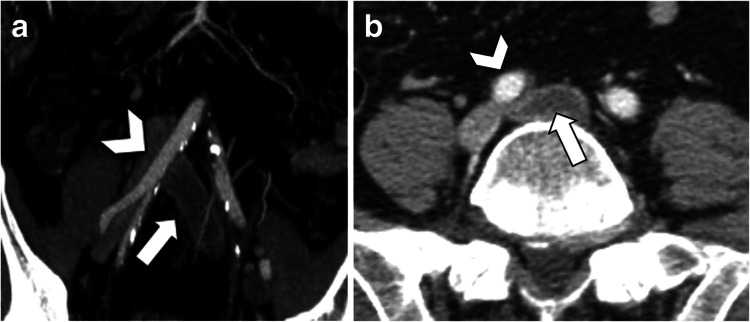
Contrast CT scan showing May-Thurner syndrome. Coronal view (a) and sagittal view (b) showing thrombosis in the compressed left common iliac vein (arrows) by the right common iliac artery (arrowheads) and the fifth lumbar vertebrae. The figure is taken from Gozzo et al. [1]. This article is licensed under a Creative Commons Attribution 4.0 International License, which permits use, sharing, adaptation, distribution, and reproduction in any medium or format, as long as you give appropriate credit to the original author(s) and the source (http://creativecommons.org/licenses/by/4.0/). CT: computed tomography.
